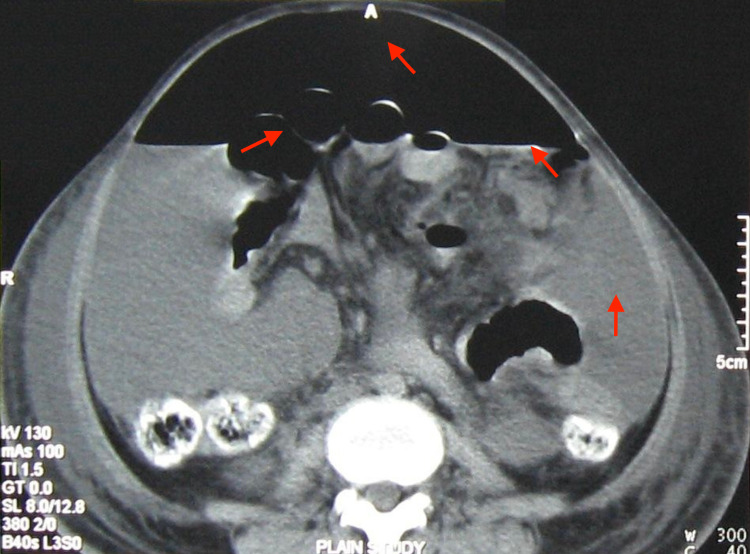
Computed tomography axial section reveals free intraperitoneal air in the anterior abdomen, diffuse ascites with air-fluid levels, and a few gas-filled small bowel loops (red arrows) TI: Time per rotation, GT: gantry tilt, A: anterior, R: right, C: center, W: window, SL: slice level.
